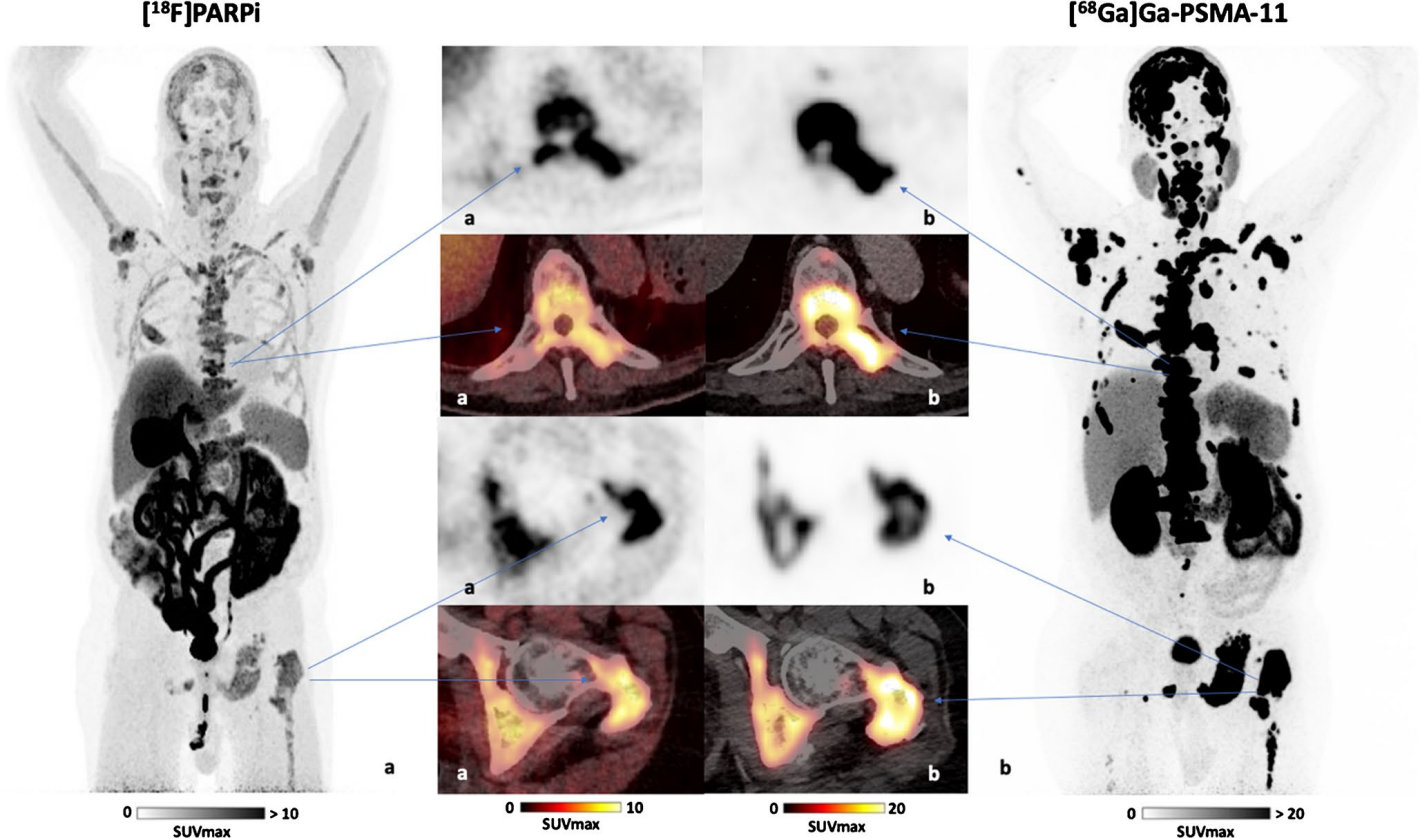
Maximum intensity projection (MIP) PET images of patient A: PET/CT images of a 63-year-old patient with metastatic castration-resistant prostate cancer (mCRPC) without a proven HRD mutation having a PSA value of 2199 ng/ml at the time of imaging. Multiple bone lesions were detected using (a) [^18^F]PARPi PET/CT, with a maximum standardized uptake value (SUV_max_) of 6.8, and (b) [^68^Ga]Ga-PSMA-11 PET/CT, with a maximum SUV_max_ >50.0
